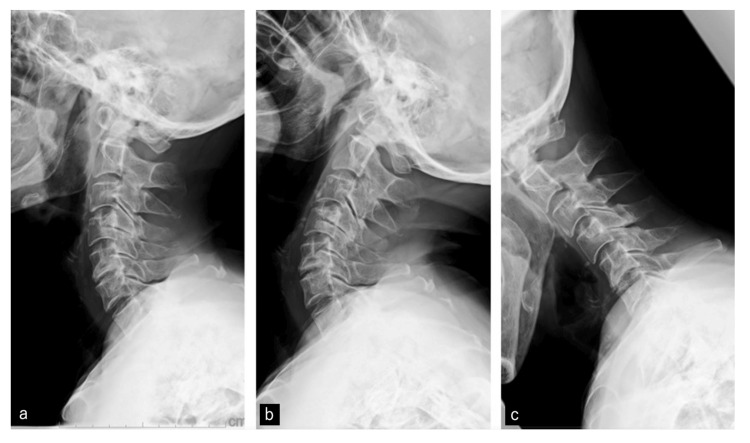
Cervical radiographs in dynamic positions (a) Neutral, (b) extension, (c) flexion. The images confirm the absence of atlantoaxial instability.
